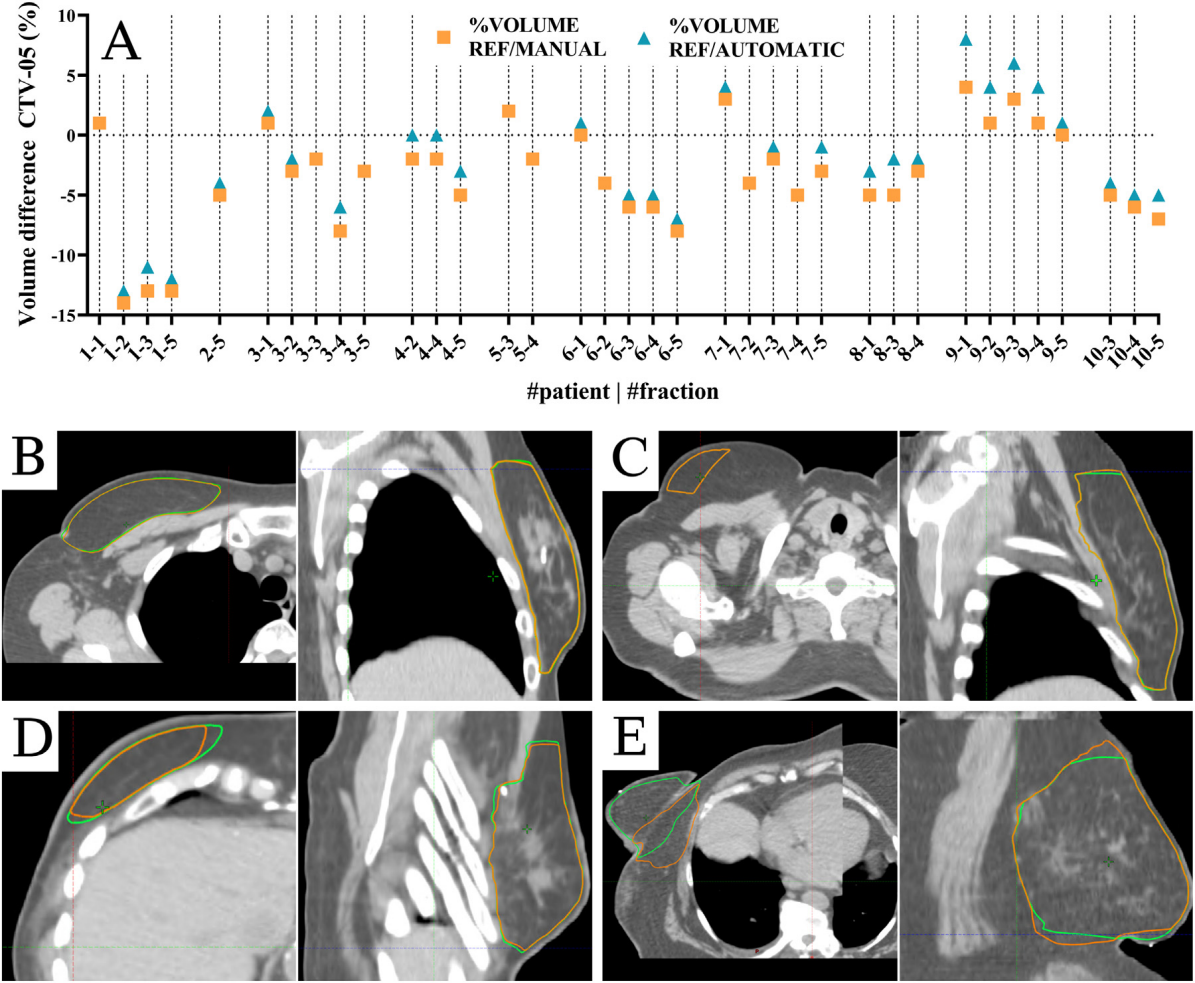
(A) Volume differences between the reference (Ref) volume divided by manually corrected volume and automatically corrected volume. (B-E) Synthetic computed tomography: orange = automatically corrected contours, and green = manually corrected contours. (B) Axial and sagittal view of patient 2 fraction 5, (C) axial and sagittal view of patient 3 fraction 2, (D) axial and sagittal view of patient 8 fraction 1, and (E) axial and sagittal view of patient 9 fraction 5. *Abbreviations:* CTV-05 = clinical target volume cropped 5 mm from the body contour.
